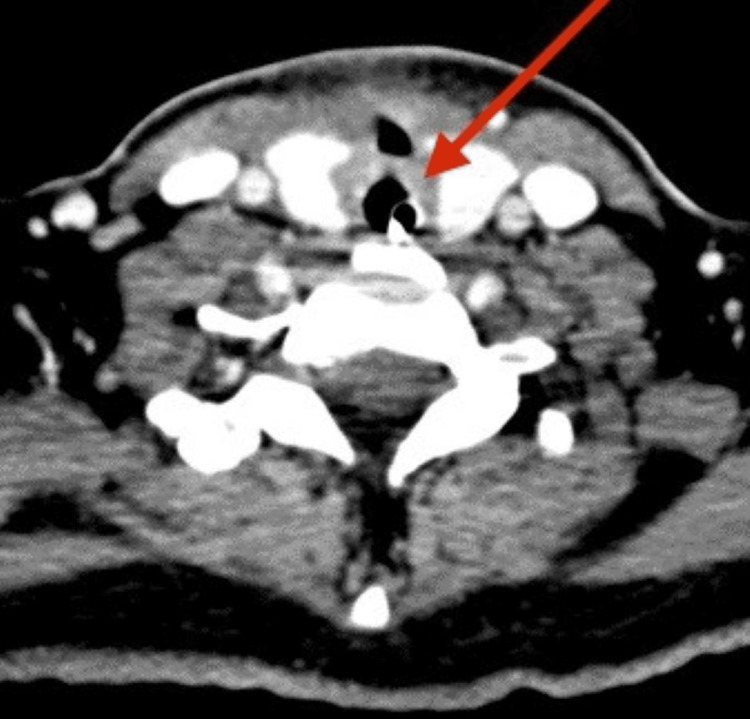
CT axial view shows the resolution of previously seen fluid along the proximal trachea (red arrow).
